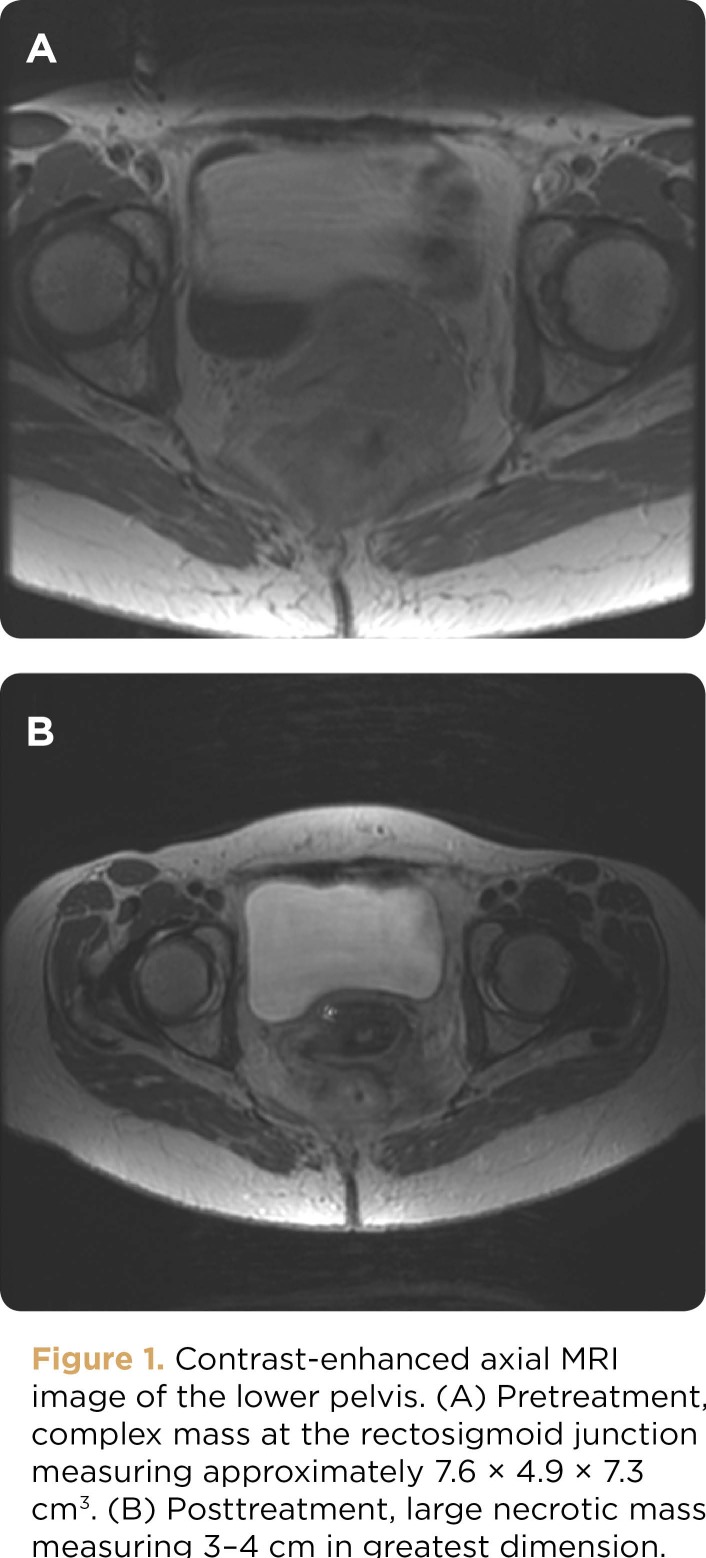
Figure 1. Contrast-enhanced axial MRI image of the lower pelvis. (A) Pretreatment, complex mass at the rectosigmoid junction measuring approximately 7.6 × 4.9 × 7.3 cm3. (B) Posttreatment, large necrotic mass measuring 3–4 cm in greatest dimension.
